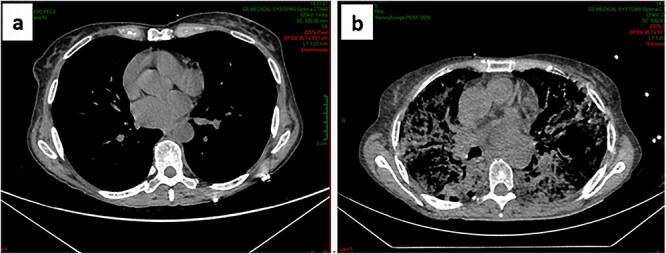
(a) Normal chest CT scan before the introduction of Gemcitabine; (b) lung scintigraphy at the onset of respiratory symptoms: diffuse bilateral infiltrate.
